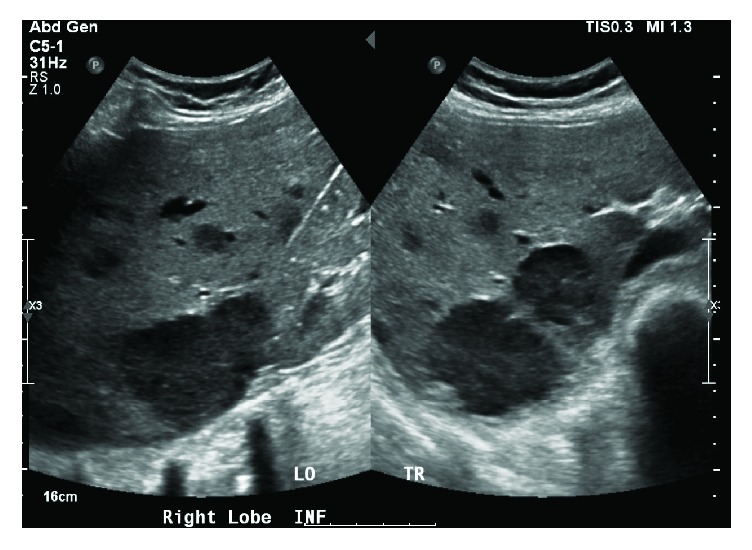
Right lobe liver ultrasound (inferior view) showing innumerable hypoechoic heterogeneous masses, the largest of which was located inferiorly and measured 6.0 × 4.3 × 5.4 cm.
